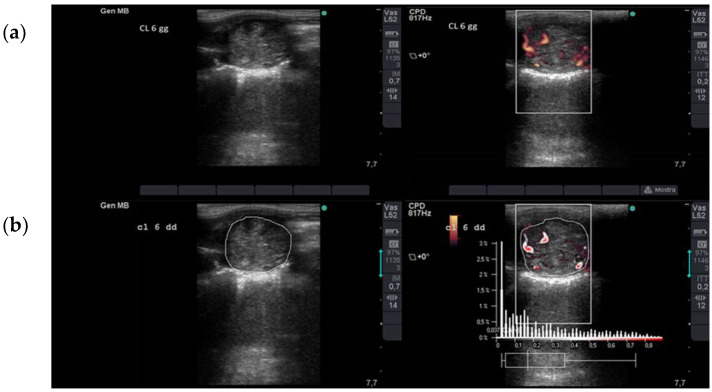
Ultrasonographic assessment of luteal blood flow. (a) B-mode (left) and Power Doppler (right) images of a corpus luteum on Day 6 after ovulation. The images are acquired at the maximum cross-sectional area of the corpus luteum. Using the Power Doppler mode, the color box is superimposed to the corpus luteum, and the blood-flow within the vessels is depicted as colored pixels. (b) Computer-assisted image analysis for semi-quantitative estimation of luteal blood flow. The corpus luteum cross-sectional area and the colored pixel area were measured using the software caliper from the B-mode (left) and Power Doppler (right) images, respectively.
